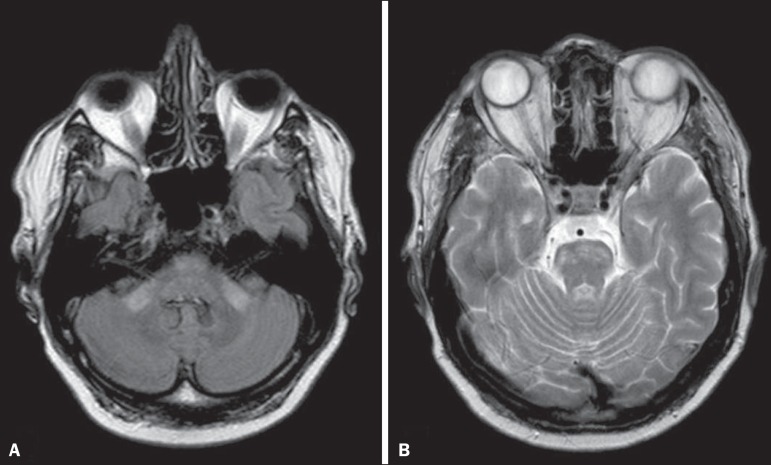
Neuro-Behçet's disease. Axial FLAIR MRI sequence
